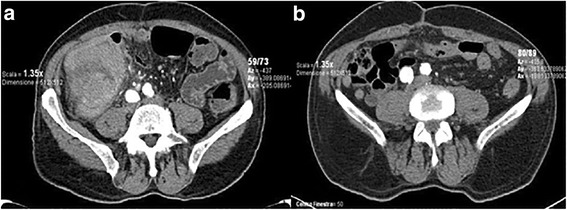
Computed tomography scan describing a mass involving cecum and ascending colon (a) and the absence of recurrence at a 4-year follow-up (b)
